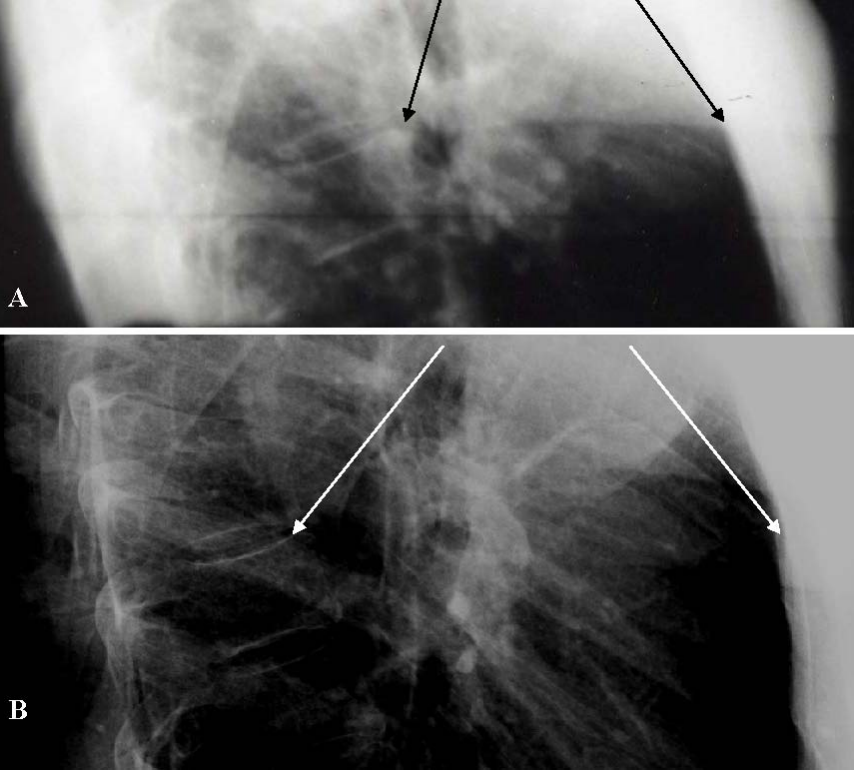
Increased sagittal plane curvature. Lateral radiographs of the thoracic spine, were taken in (A) 2001; and (B) 2005. Arrows indicate the anterior edge of T7 (left) and a bony landmark on the posterior edge of the sternum (right). Radiographs were taken by the same technician, with the patient in a standing position, at maximum inhalation, using the same machine (University Medical Center, Tucson AZ).
